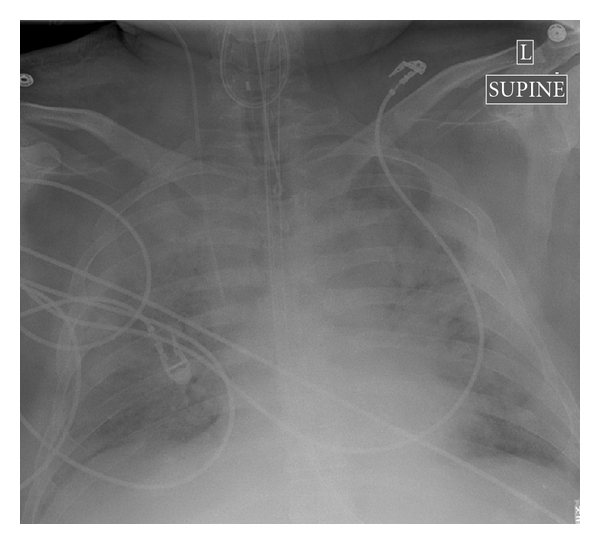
Admission chest X-ray showing severe bilateral diffuse pulmonary infiltrates.
